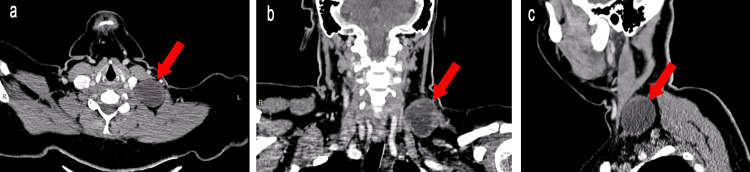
Contrast CT of neck a) Axial view showing a well-defined cystic lesion on the left side abutting the carotid vessel; b) Sagittal view showing the lesion abutting the paraspinal muscles; c) Coronal view showing a lesion at the root of the neck
